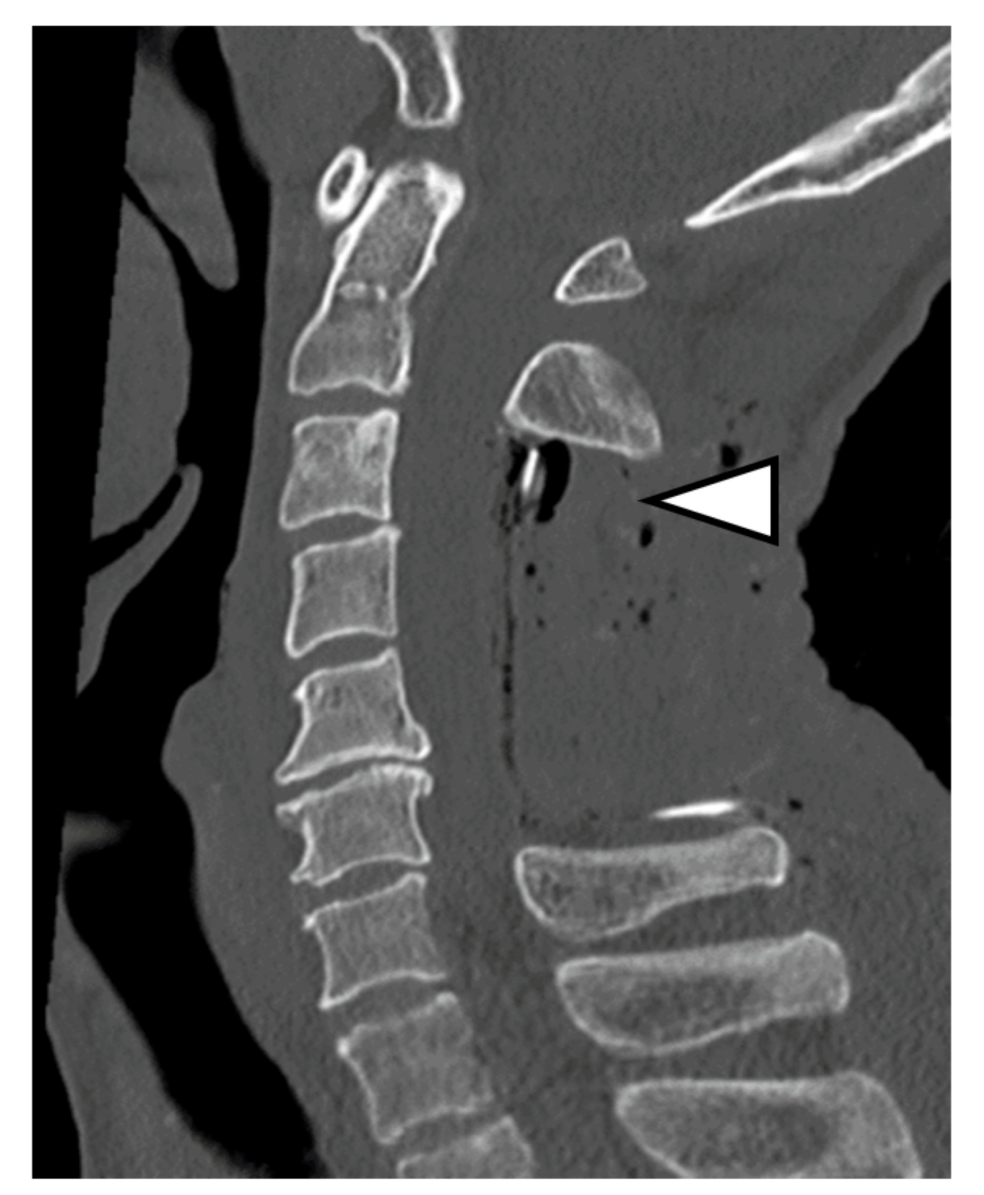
Post-reoperative cervical spine CT (sagittal image) The C3 lamina was completely resected (arrowhead) CT: computed tomography
